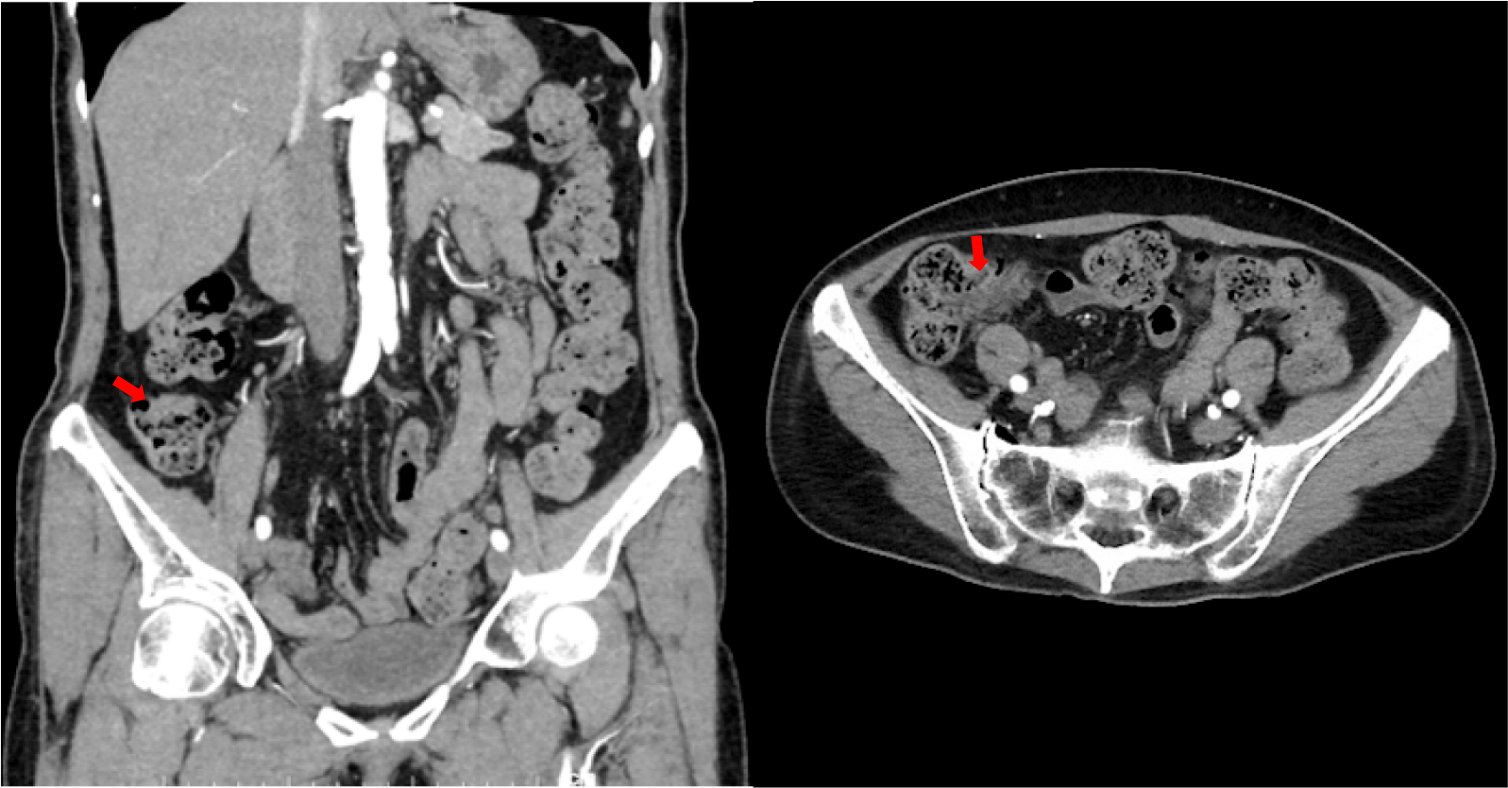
Abdominal contrast-enhanced CT images. CT revealed wall thickness at the ascending colon (red arrow)
